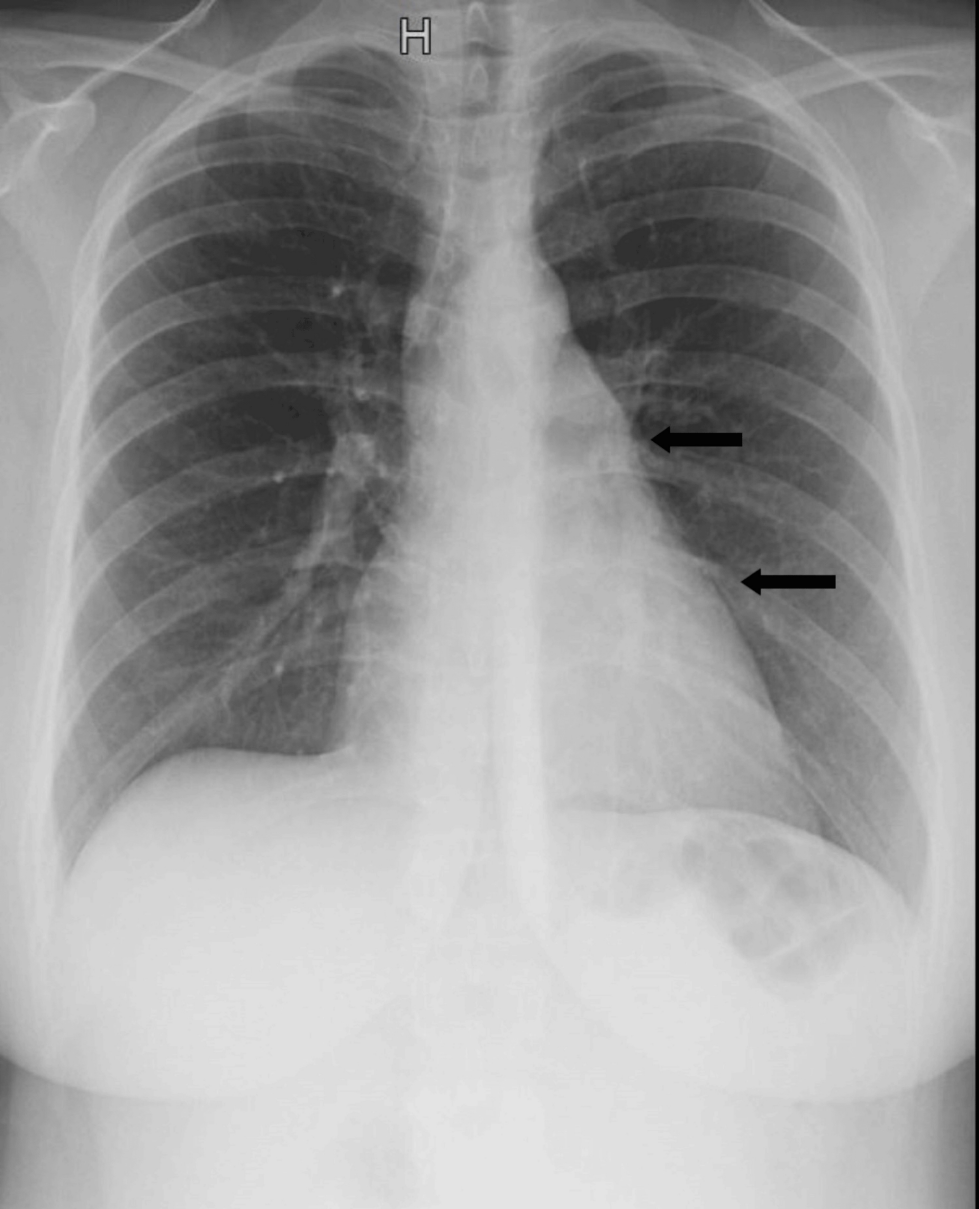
Chest X-ray with the typical appearance of mediastinal pneumothorax A thin, clear border is seen surrounding the cardiac silhouette and thoracic distension, consistent with air accumulation in the mediastinum.
